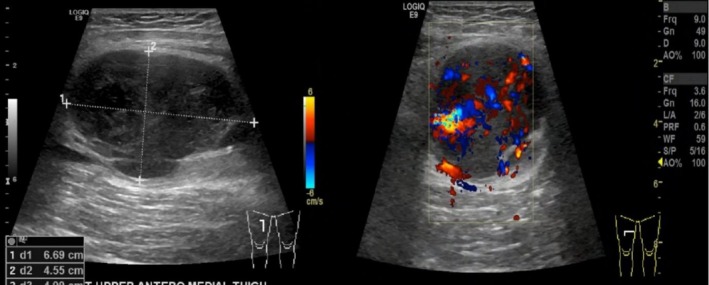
Ultrasound soft tissue: Well‐defined, hypoechoic, encapsulated lesion with internal vascularity, measuring 6.6 × 4.5 × 4.9 cm, consistent with a pseudoaneurysm.
